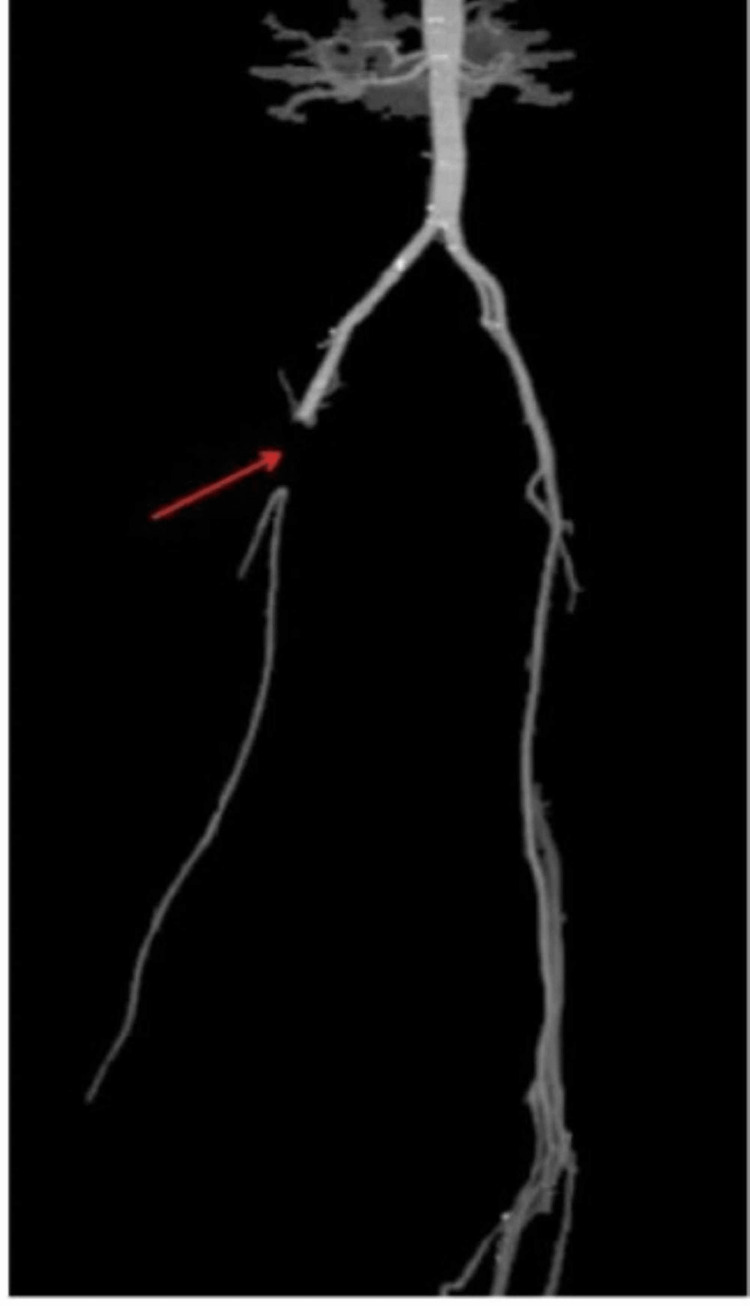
CT Angiogram (CTA) showing abrupt disruption of the common femoral artery (CFA) (red arrow).
